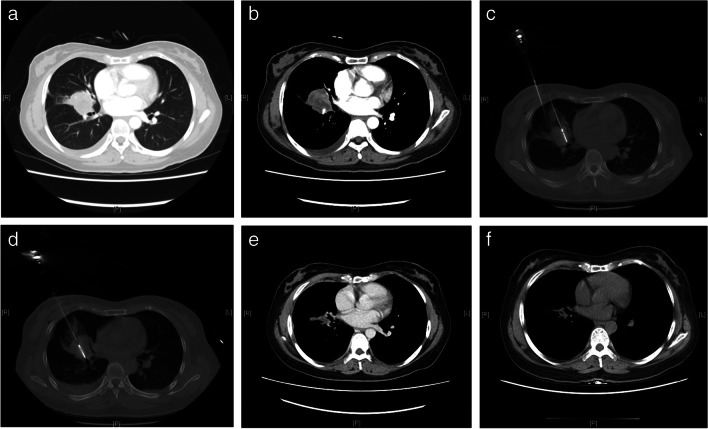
Imaging data of a typical case. a CT conducted before MWA. b Contrast-enhanced CT conducted before MWA. c, d CT during MWA. Two antennae were used. e Contrast-enhanced CT conducted 1 year after MWA. f Contrast-enhanced CT conducted 2 years after MWA
